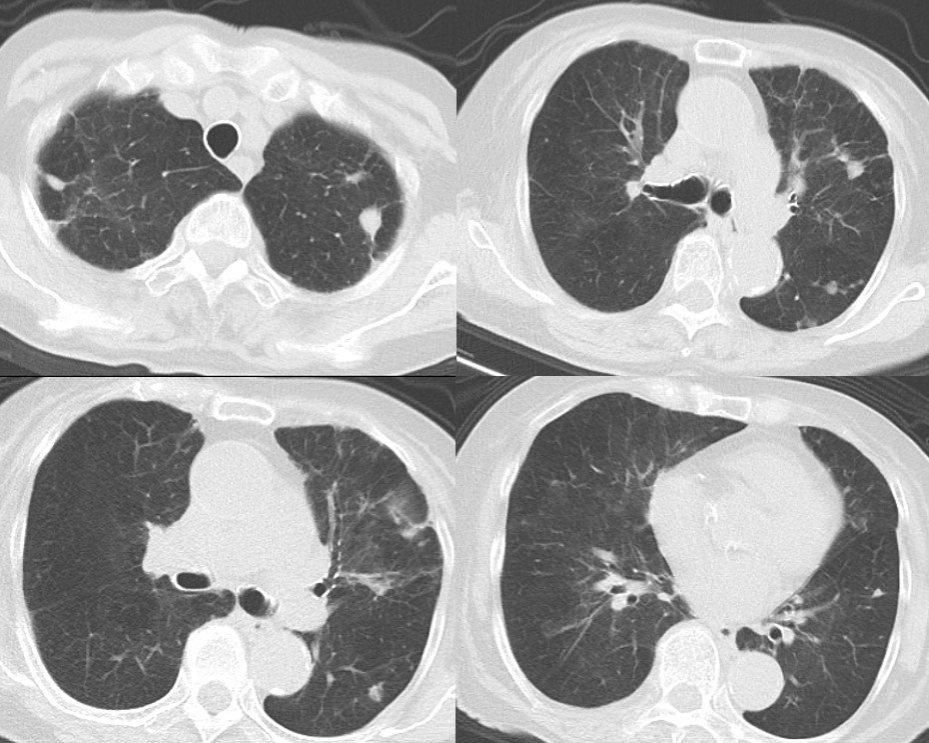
A CT scan of the chest performed 2 years after treatment. The pulmonary nodules and consolidation had resolved. Slight pulmonary scarring remained.
